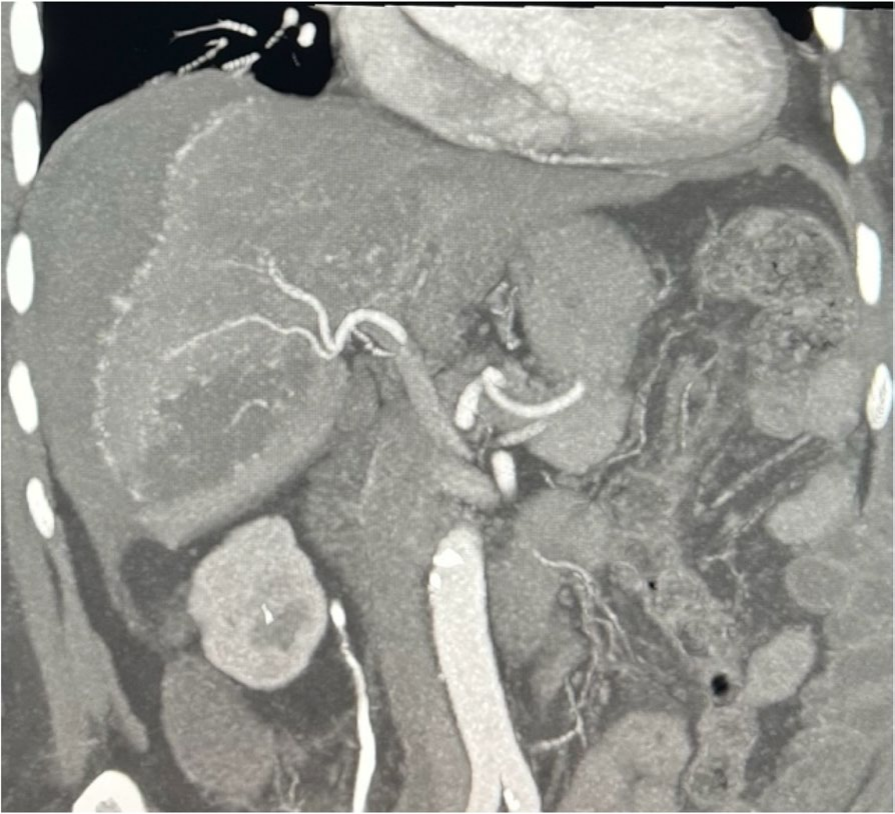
Coronal maximum intensity projection (MIP) arterial phase CT liver showing multiple abnormal vessels at the capsular surface of the right hepatic lobe
